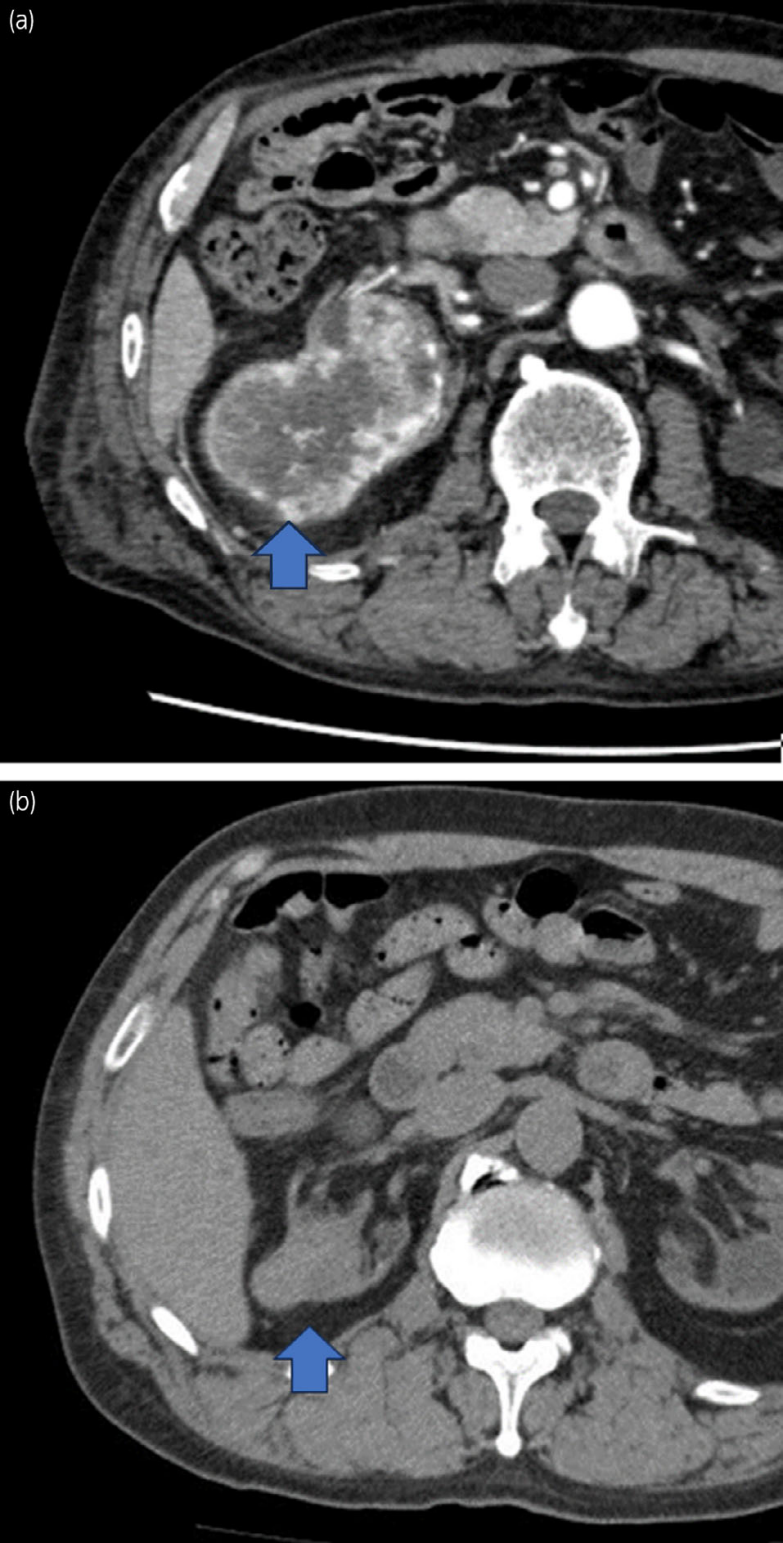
(a) Computed tomography image at admission showing a mass with fresh hematoma in the upper pole of the right kidney (blue arrow). (b) Computed tomography from 8 years earlier showing a 40‐mm internally heterogeneous mass in the upper pole of the right kidney (blue arrow).
